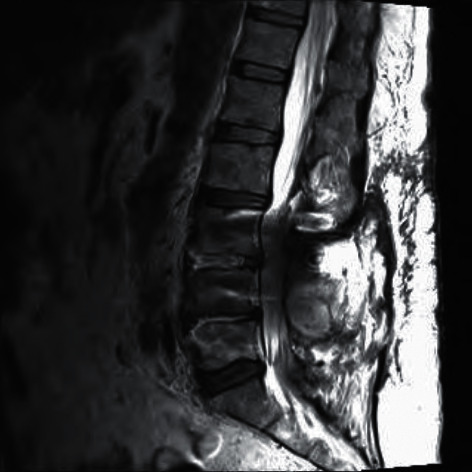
MRI of the lumbar spine revealing osteomyelitis at the L4-L5 level.
